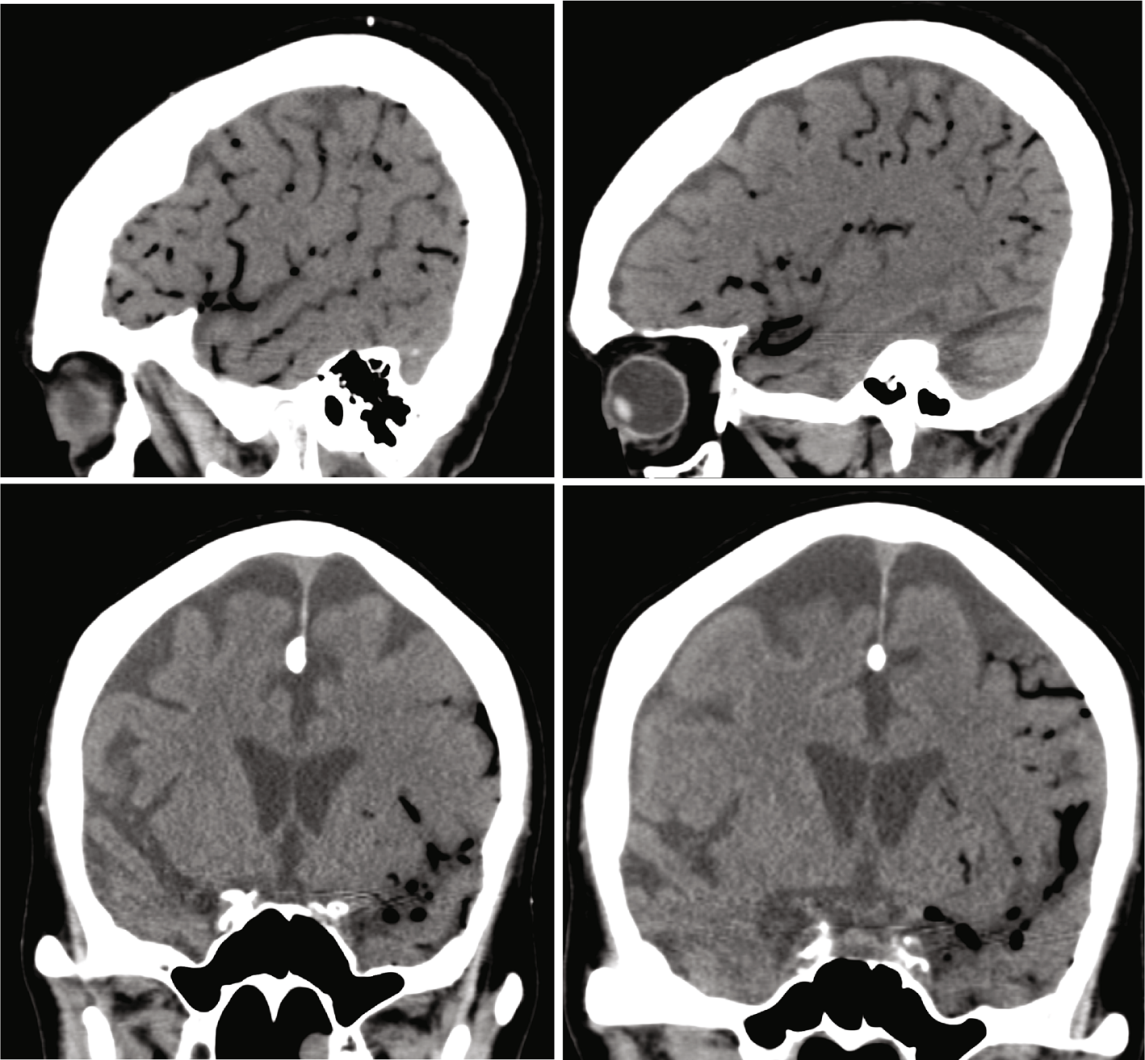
Sagittal and coronal CT images of the head without contrast demonstrate intravascular air within the left MCA distribution.
